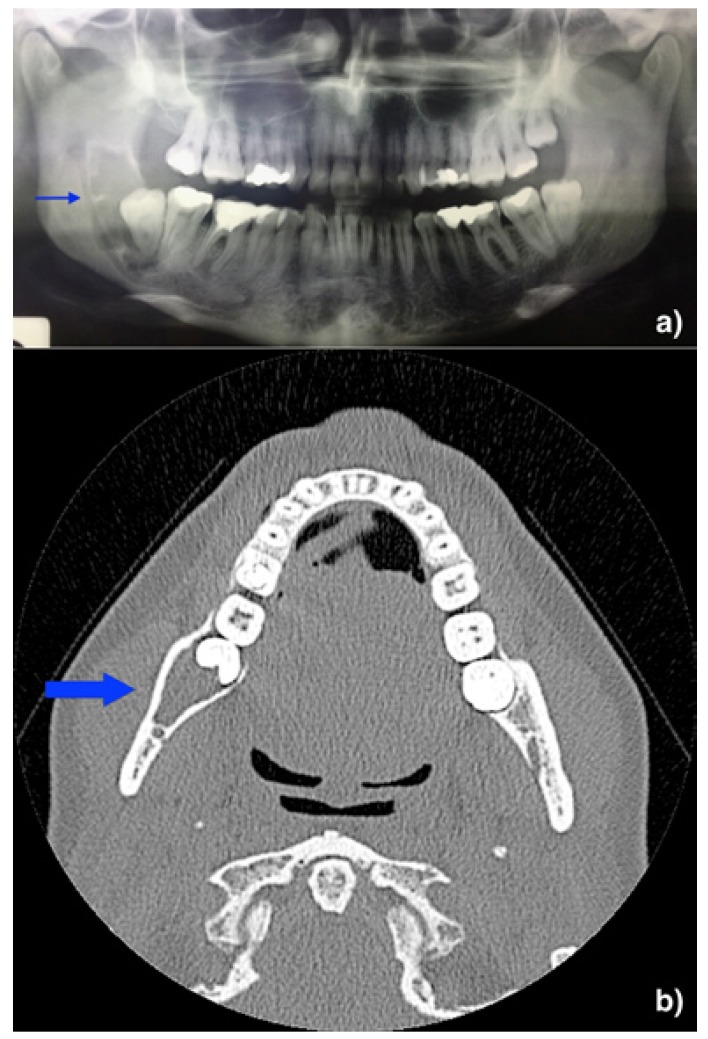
Radiographic imaging – A) orthopantomography showing a radiolucent multilocular and multilobular lesion associated with impacted right third molar of the mandible. B) axial CT Scan showing the radiolucent lesion within intact buccal and cortical bone.
